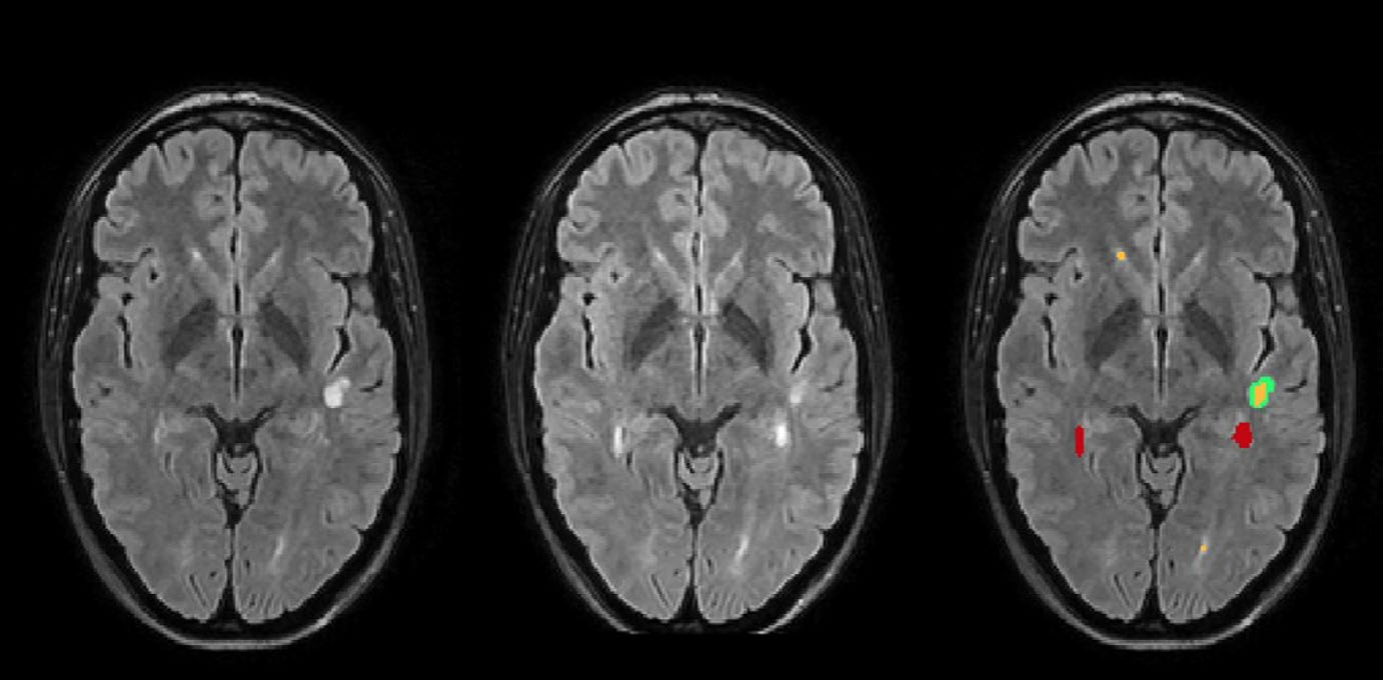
An example of LST's comparison of two time points is shown for one patient. Axial slices of FLAIR images superimposed with white matter lesion maps are shown: left, time point 1; middle, time point 2; right, white matter lesion changes with the following color coding: red, increased; yellow, unchanged; green, disappeared. ml, milliliter
